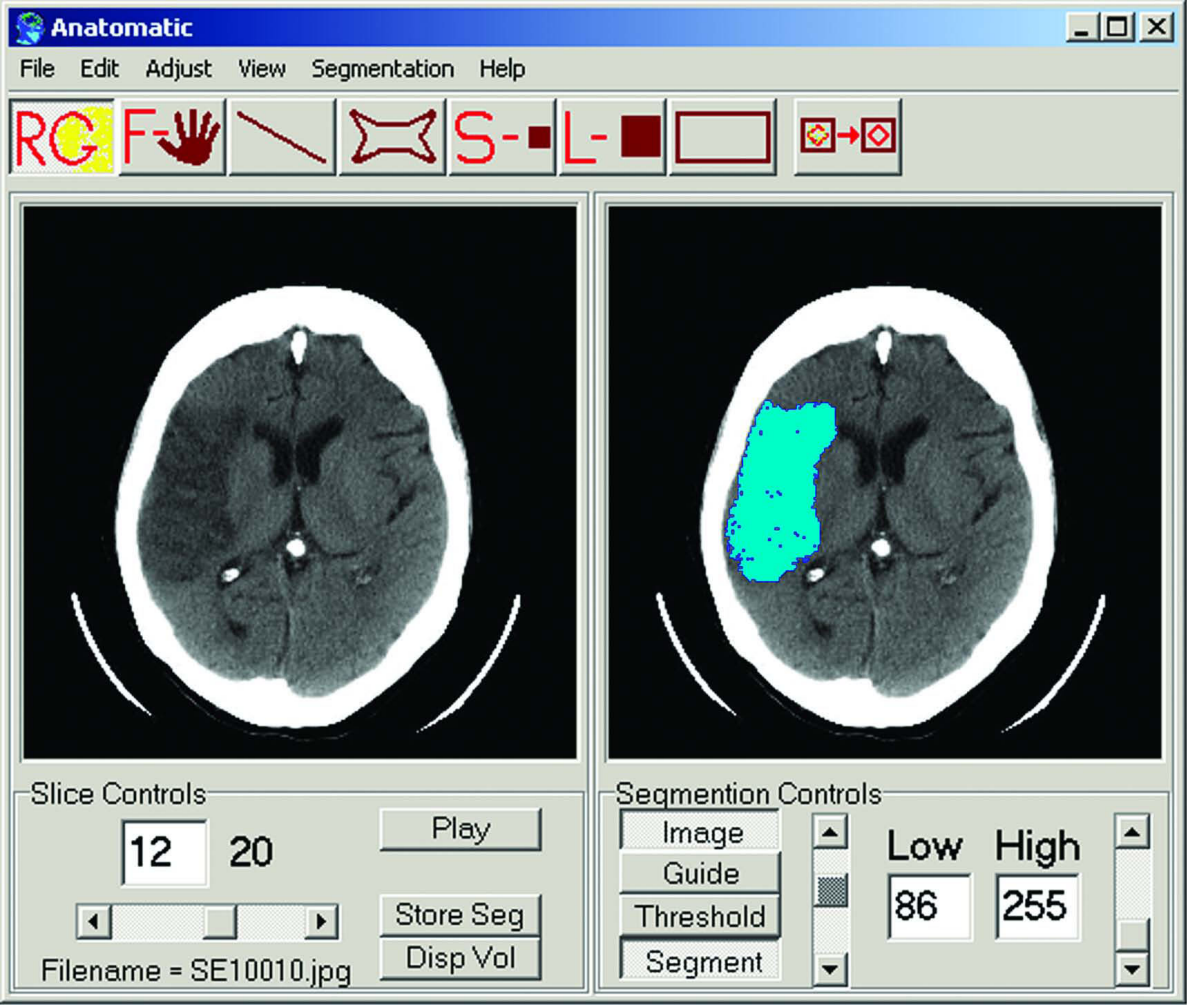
Software for volumetric analysis. The user interface of Anatomatic™ 2.23 software was used in the volumetric analysis. The figure on the left represents the original axial CT slice depicting a large temporal lobe infarction. The figure on the right represents the accurately segmented area of infarction.
